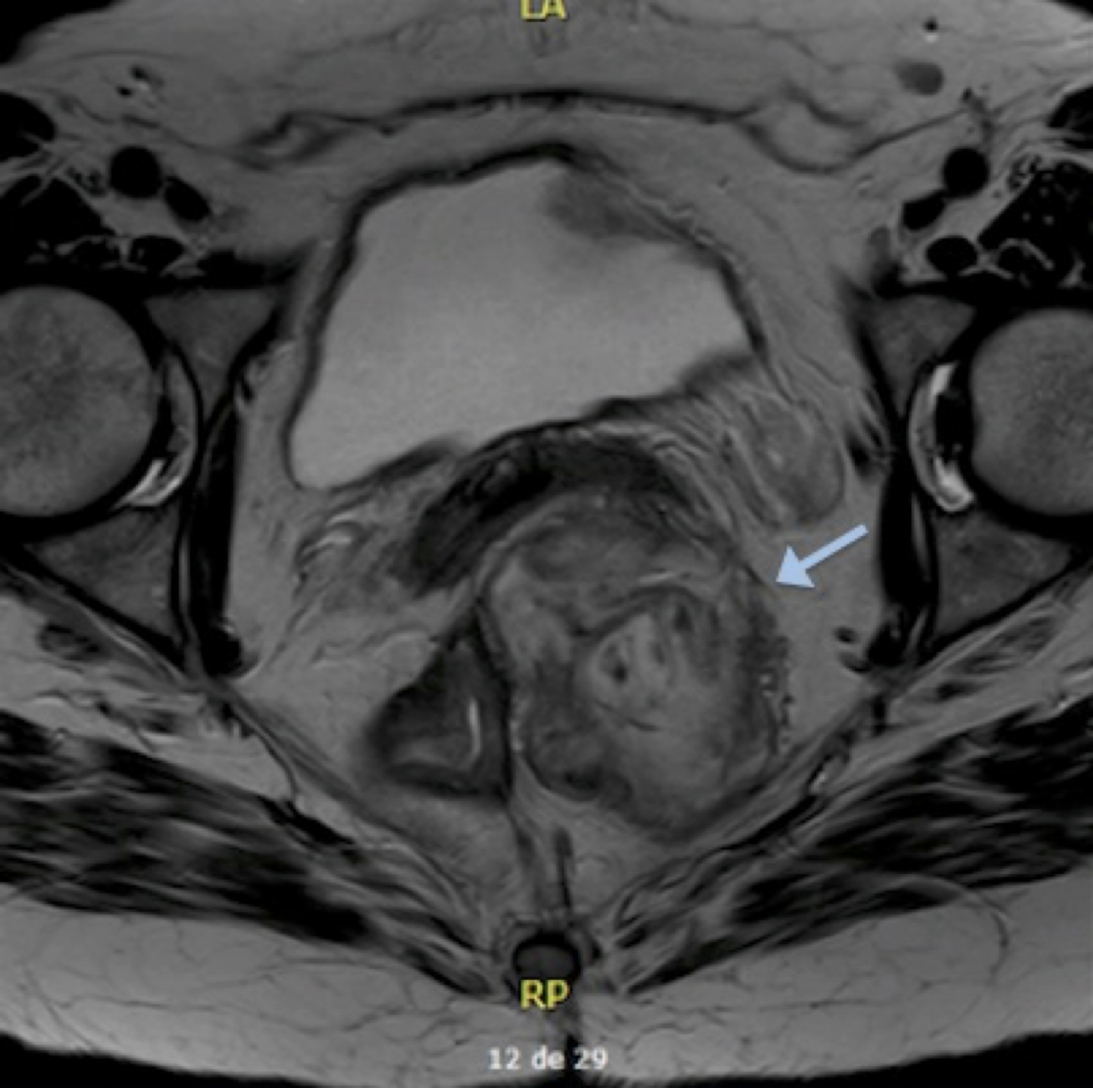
Pelvic magnetic resonance imaging Left perirectal mass pushing the rectum slightly to the right
